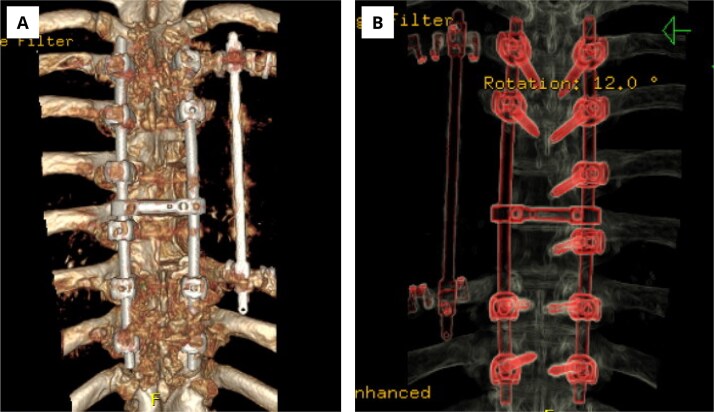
Postoperative computed tomography of the thoracic backbone with three-dimensional reconstruction.
